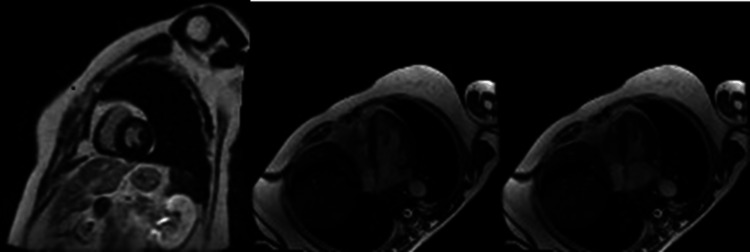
Cardiac MRI Cardiac MRI showed mild left ventricular hypertrophy. The right ventricle had no abnormality. MRI: magnetic resonance imaging
